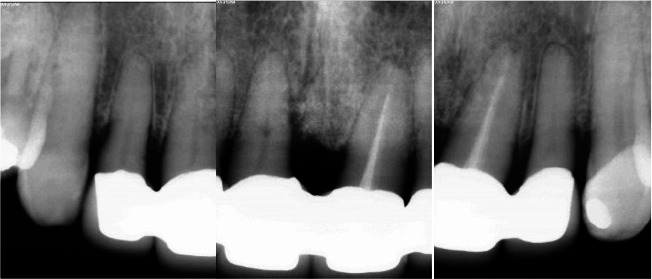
Radiographic evaluation 12 years post-surgery, no change in bone aspect or volume is noticed
